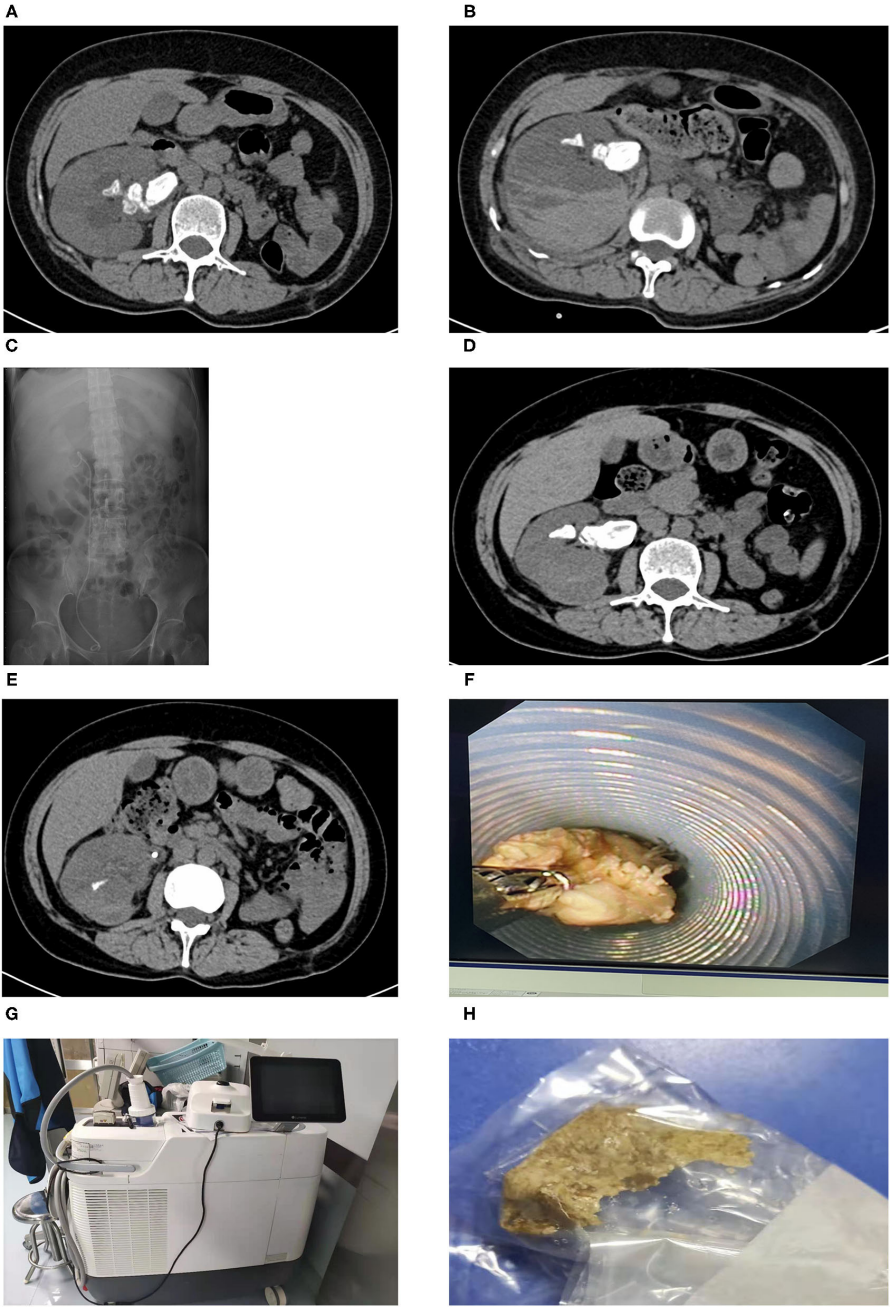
The CT images throughout the period: (A) The abdominal CT at admission showing increased right kidney and staghorn stones among which the larger stone was about 3.0 × 1.8 cm; (B) the abdominal CT after PCNL of right renal stones, which was terminated due to severe bleeding during channel establishment; (C) the abdominal KUB film after right ureteral stent placement; (D) the abdominal CT at readmission; (E) the postoperative abdominal CT of the third flexible ureteroscopy; (F) the image of flexible ureteroscopy forceps grabbing floppy stone; (G) Lumenis Pulse 120H; (H) infectious stones after the third procedure. PCNL, percutaneous nephrolithotomy.
